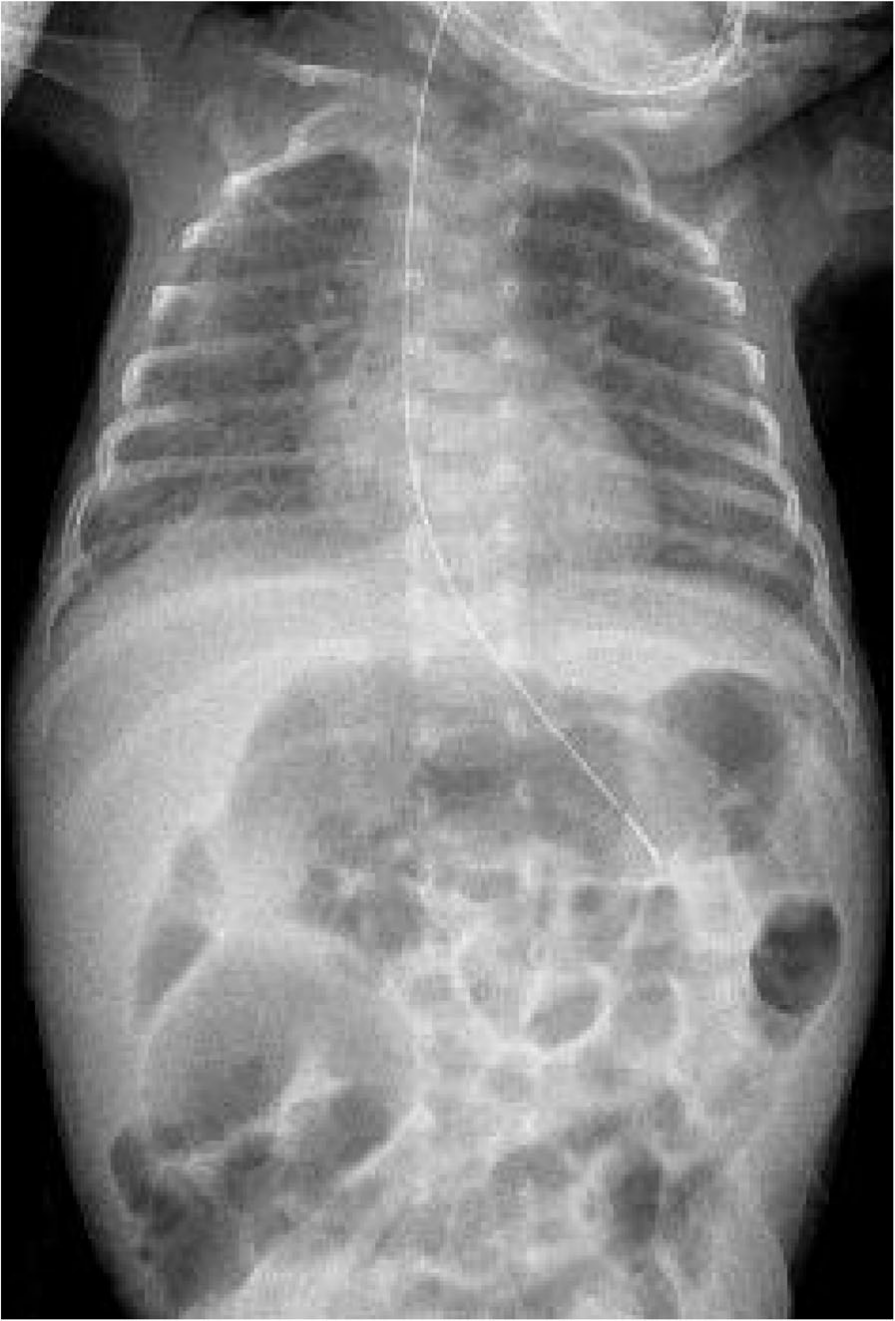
Chest x-ray at discharge. Chest x-ray at 78 day of life showing the disappearance of the fibrin glue
